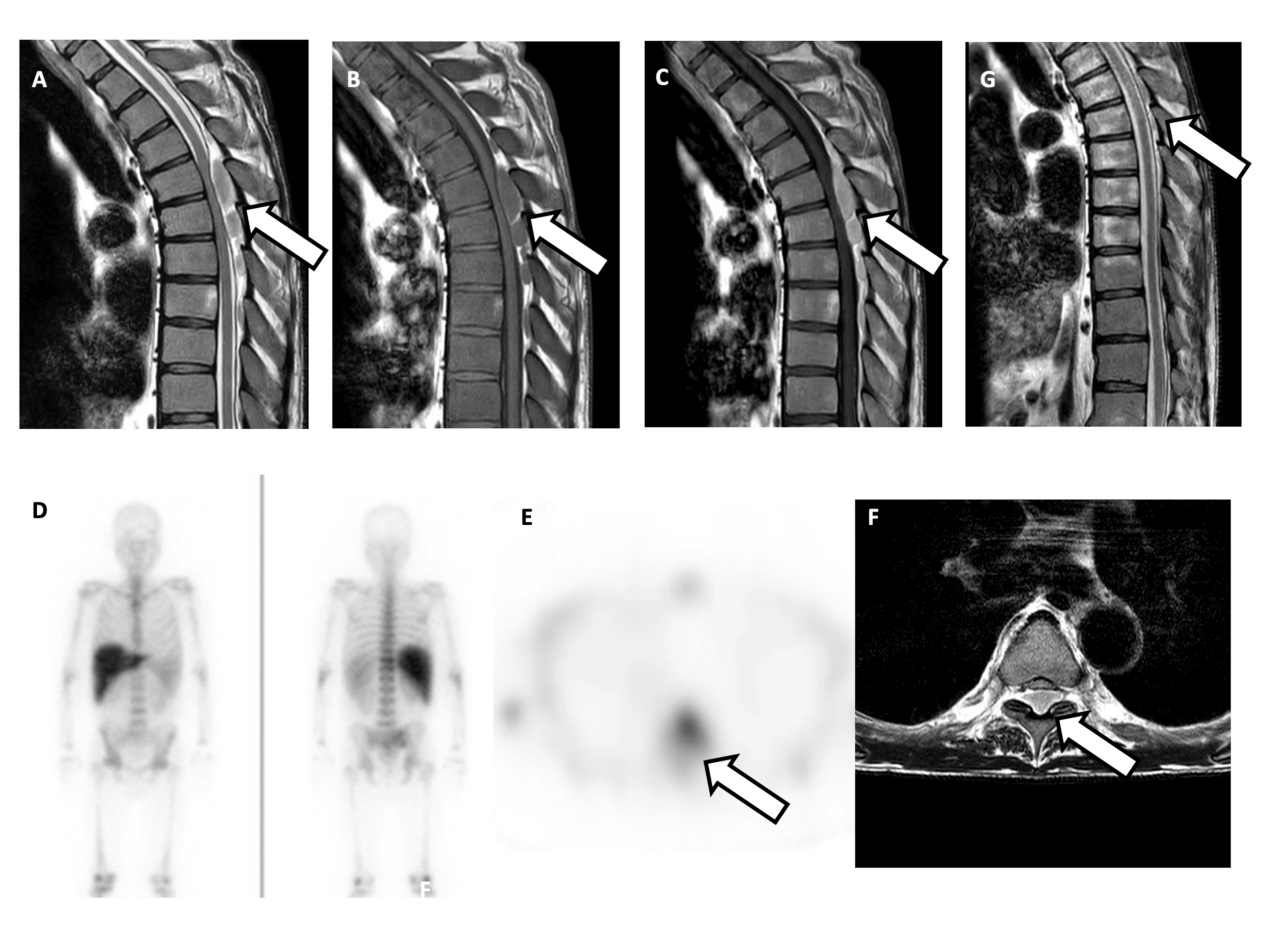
A-G:MRI and In-111 bone marrow scintigraphy A-C: The posterior epidural mass within the spinal canal compresses the dural sac and causes spinal cord compression, as indicated by the arrows. D-F: Although not visualized on planar bone marrow scintigraphy, faint radiotracer accumulation corresponding to the mass is observed on SPECT imaging, marked by arrows. G: The epidural mass decreased in size following radiation therapy, as shown by the arrow. A: T2WI sagittal view, B: T1WI sagittal view, C: Gd-T1WI sagittal view, D: ^111^In bone marrow scintigraphy planar imaging, E: ^111^In bone marrow scintigraphy SPECT imaging, F: T2WI axial view, G: Post-treatment T2WI sagittal view. Abbreviations: Gd-T1WI, gadolinium-enhanced T1-weighted image; T1WI, T1-weighted image; T2WI, T2-weighted image; SPECT, single-photon emission computed tomography
